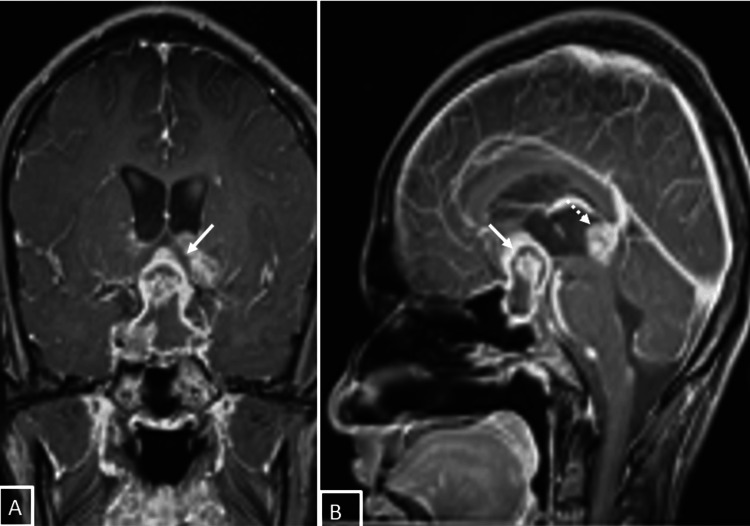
MRI of the brain (A) coronal and (B) sagittal post-contrast images showed a heterogeneously enhancing lesion involving the sella and suprasellar region (bold arrow) and another similar lesion in the pineal gland (dotted arrow).
